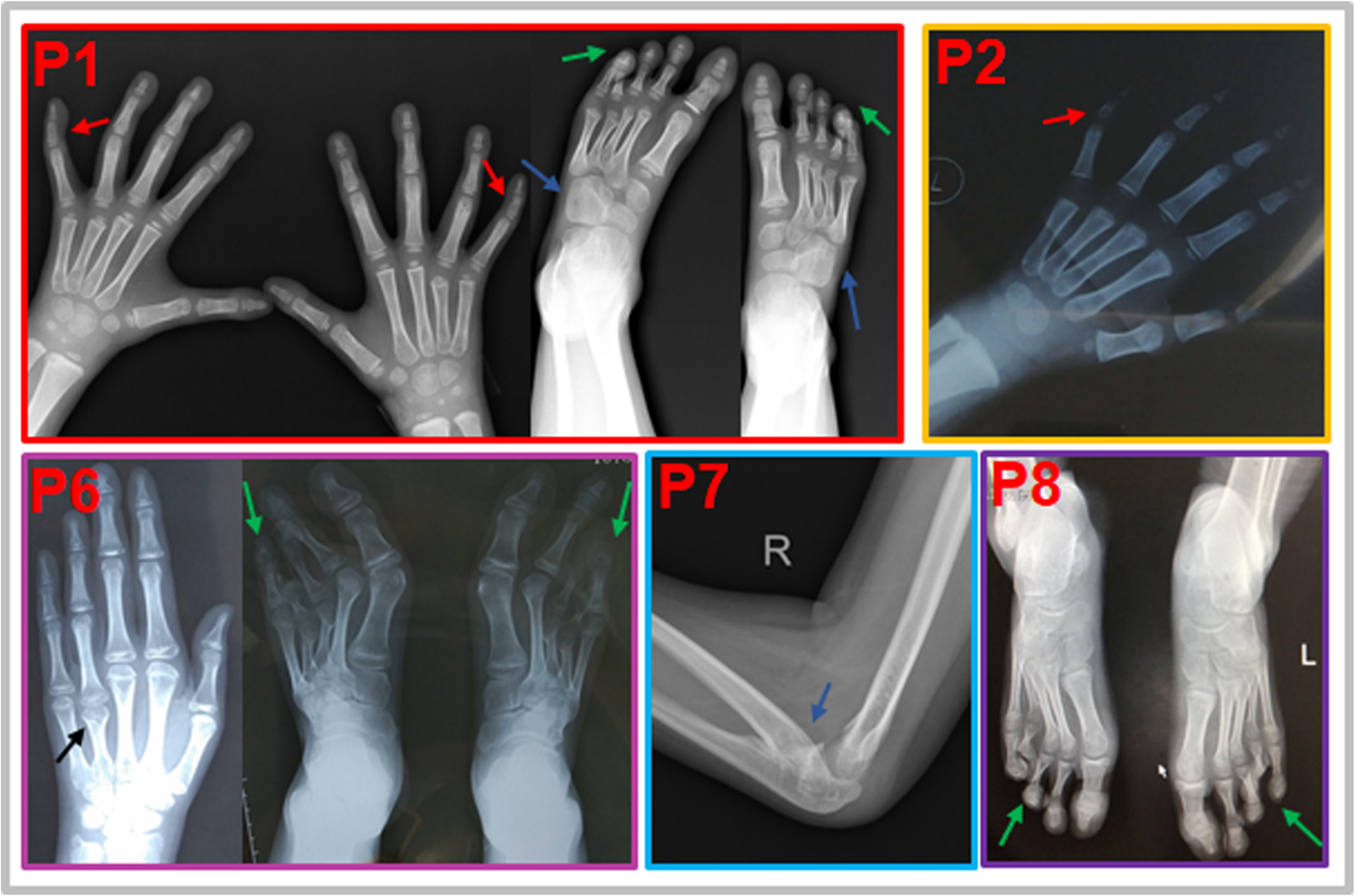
The skeletal X-ray results of Chinese children with ABS caused by PORD. Notable skeletal abnormalities included clinodactyly of the 5th fingers (red arrow), brachydactyly (green arrow, short distal phalanges for patient 1; short 3rd and 4th toes for patients 6; short 4th toes for patients 8), short 4th metacarpals (black arrow), and abnormal bone fusion (blue arrow, fusion between the lateral cuneiform bone and cuboid bone for patient 1; radioulnar synostosis for patient 7)
